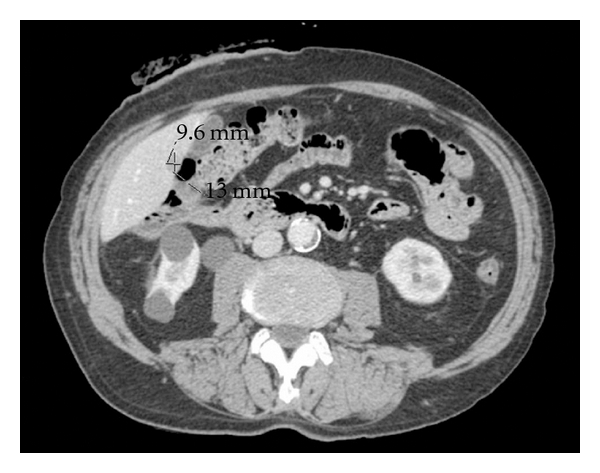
CT scan showing the metastasis located in segment 5, in close relation to the gall bladder.
